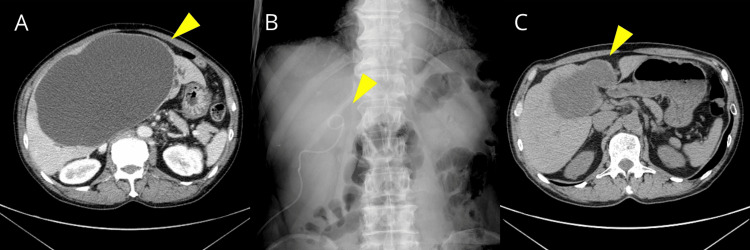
Example of clinical response (A) Axial abdominal CT: Image showing a simple hepatic cyst prior to percutaneous aspiration sclerotherapy. (B) Fluoroscopic image: Image showing placement of a pigtail catheter and instillation of MINO during the sclerotherapy procedure. (C) Axial abdominal CT: Image obtained one year after treatment, demonstrating a reduction in cyst volume to less than 50%. MINO, minocycline hydrochloride
